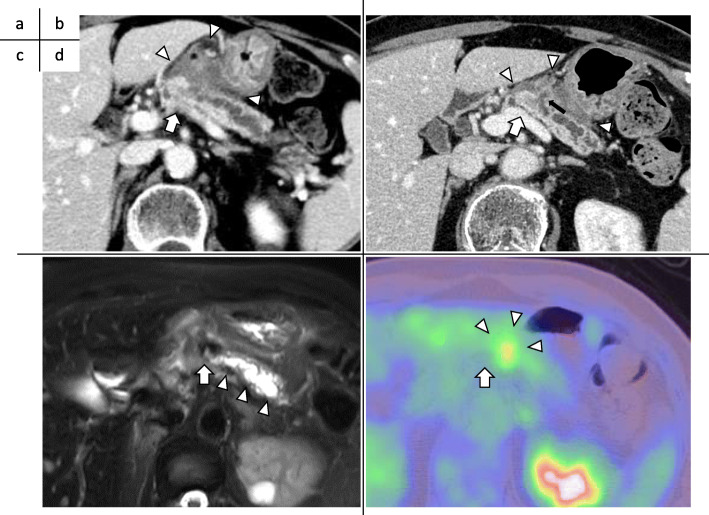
Imaging findings. a Abdominal multidetector-row computed tomography (MDCT) at the referral hospital. A hypovascular tumor was present in the body of the pancreas (arrow), and the distal pancreatic duct was dilated. In addition, a slightly low-density area (LDA) was found to be spread over the ventral side of the pancreas (arrowhead) and touching the stomach. b MDCT images obtained 2 months later at our hospital. There was no significant change in the tumor size (arrow) or pancreatic duct dilatation, but the LDA at the ventral side of the pancreas had shrunk (arrowhead). The two-tone duct sign and cork-of-wine-bottle sign were observed (arrow). Retrospective examination revealed findings that seemed to indicate the rupture of the main pancreatic duct (MPD) and the formation of a fistula extending outside of the pancreas (black arrow). c T2-weighted magnetic resonance image taken at our hospital. Constriction of the MPD (arrow) and distal MPD dilatation (arrowhead) was observed, but the tumor was unclear. d Positron emission tomography-computed tomography taken at the referral hospital. Weak accumulation of fluorodeoxyglucose was present at the ventral side of the pancreas, corresponding to the LDA on MDCT (arrowhead); however, no apparent accumulation was noted in the tumor (arrow)
